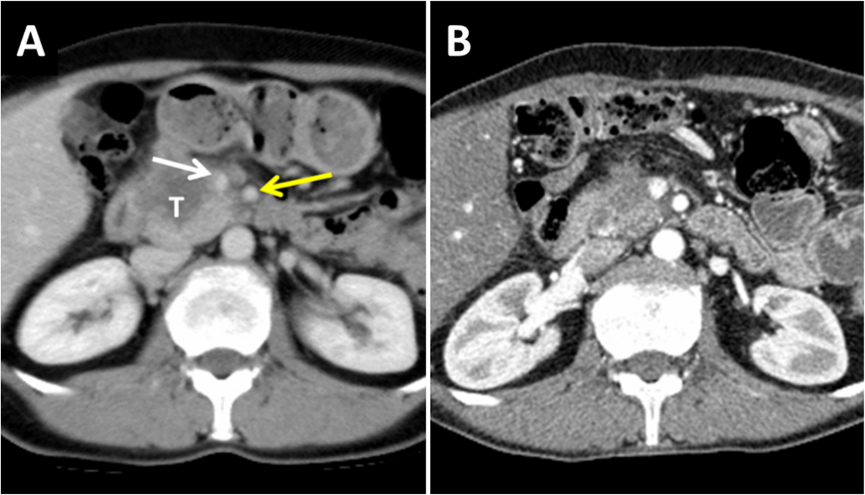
Computed tomography (CT) scan of patient number 2 before the initial palliative bypass procedure (A) showing a huge tumor of the head of the pancreas (T) with tumoral infiltration of both superior mesenteric vein (SMV) (white arrow) and superior mesenteric artery (SMA) (yellow arrow). Following six cycles of FOLFIRINOX chemotherapy and bypass procedure, the CT scan showed regression of the infiltration of the SMA with no detectable metastases (B).
